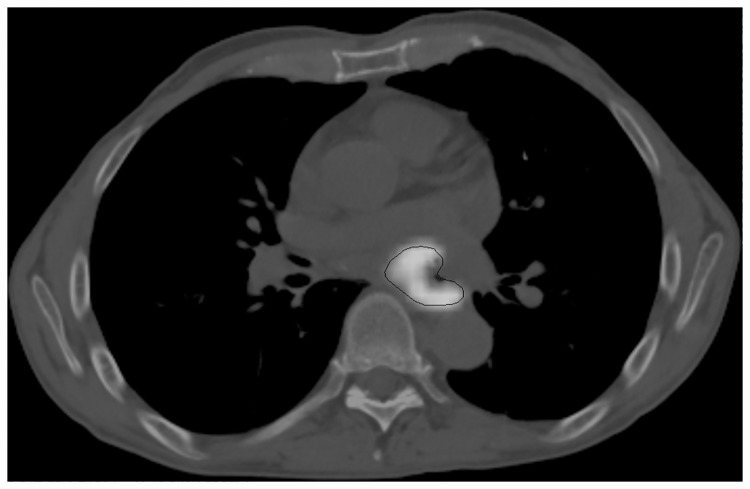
PET/CT slice of the chest with a segmented esophageal tumor.
